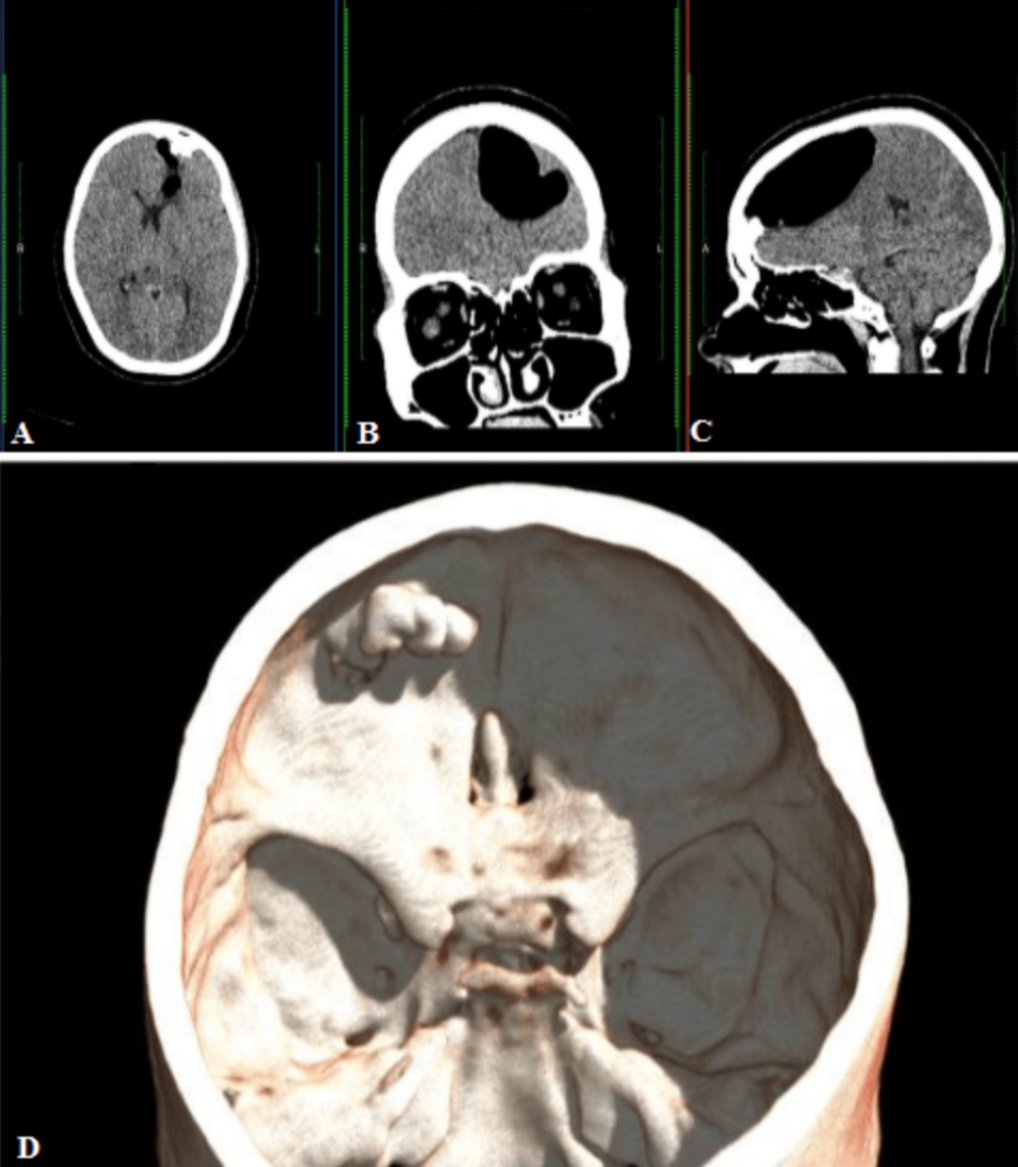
Non-enhanced CT brain axial (A), coronal (B), and sagittal (C) images showing a homogeneous bony mass within the left frontal sinus with associated pneumocephalus. 3D reconstruction image (D) showing the left frontal sinus osteoma from the superior aspect CT: computed tomography, 3D: three dimensional
